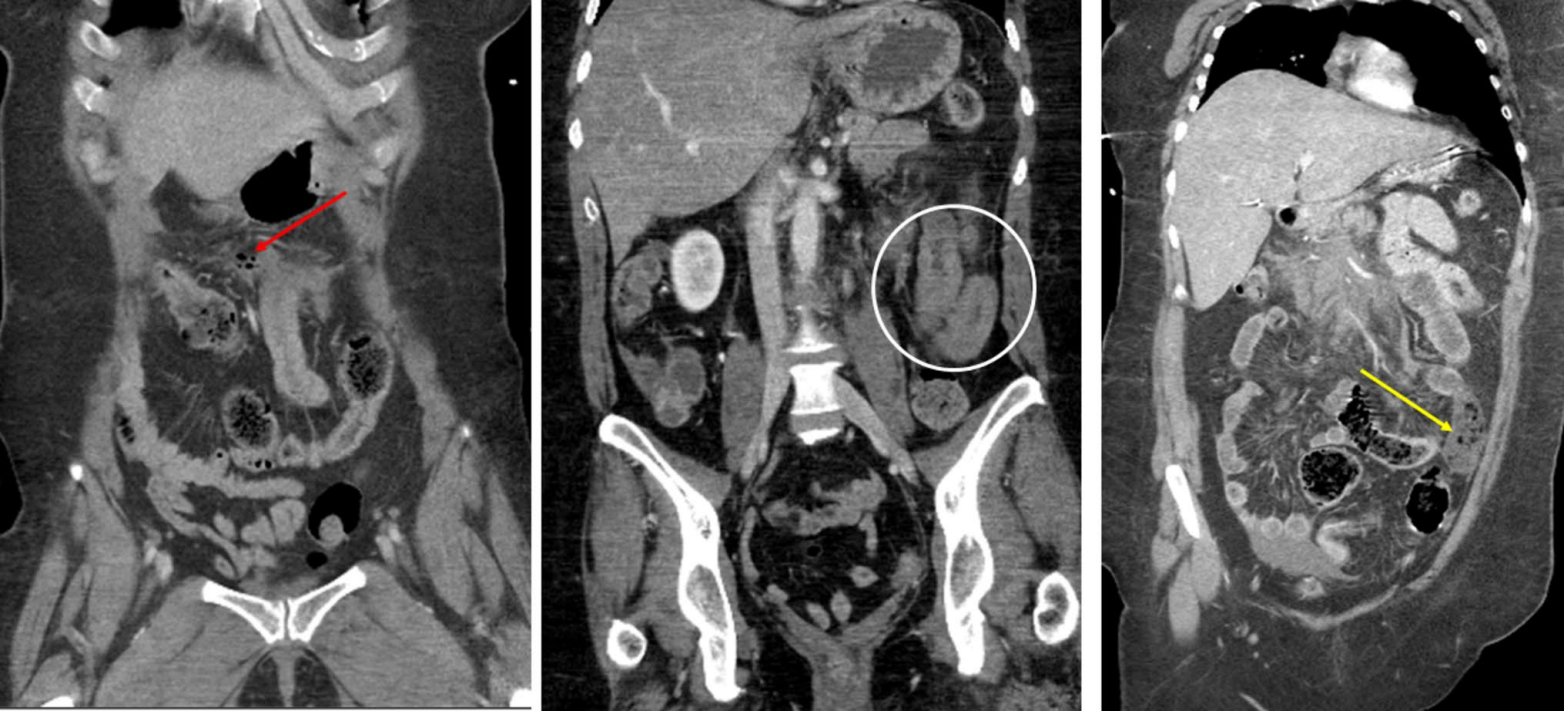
(Left) Coronal CT of the abdomen showing a collection of free air and fluid adjacent to a small bowel loop (red arrow). (Center) Coronal CT of the abdomen in a different patient showing bowel wall thickening with interloop fluid collection (white circle). (Right) Coronal CT of the abdomen in a different patient showing a devascularized bowel (yellow arrow)
